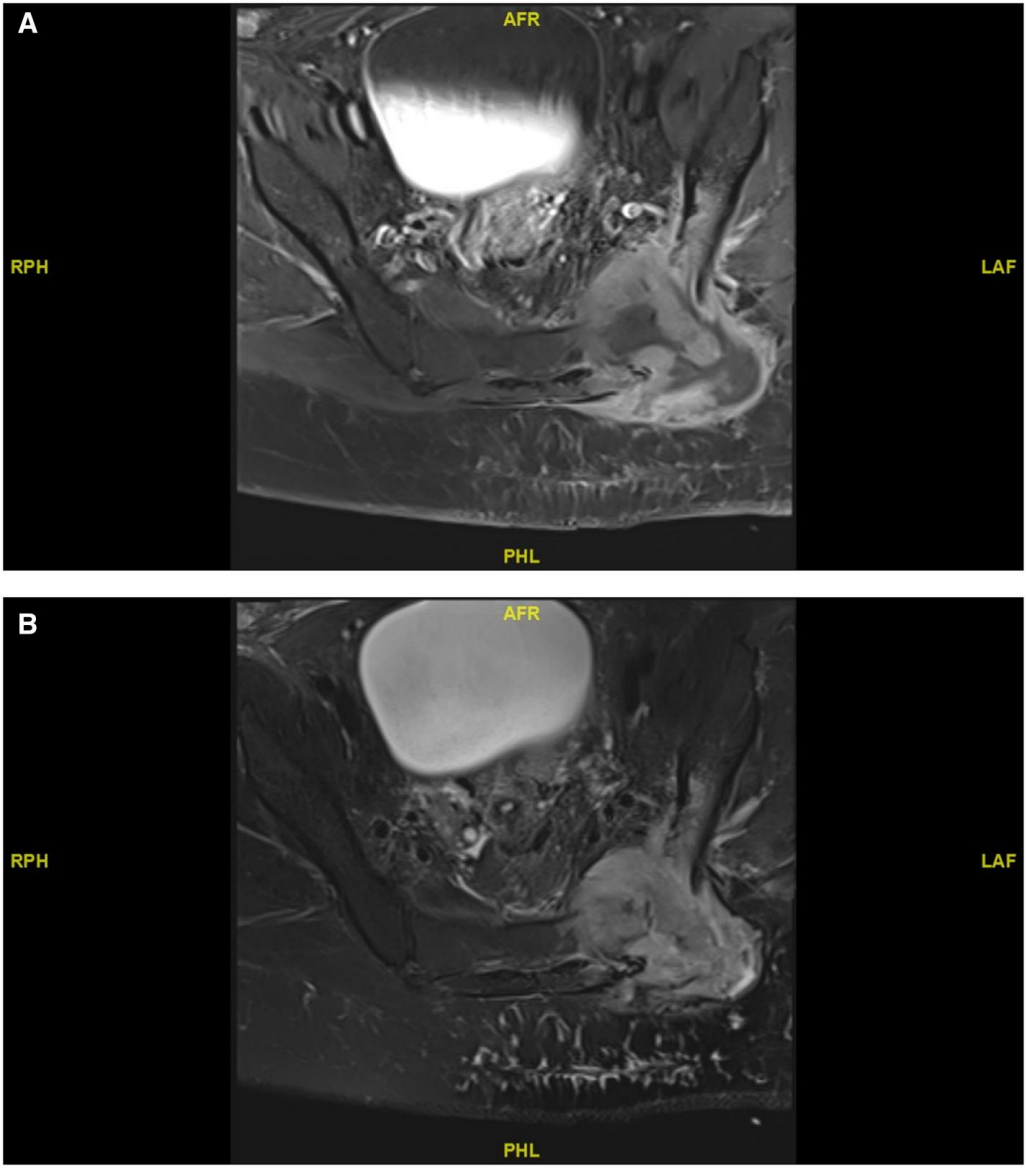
MRI axial (A) T1-weighted and (B) T2-weighted image showing osteomyelitis and cellulitis in the left gluteal region of the lower back.
